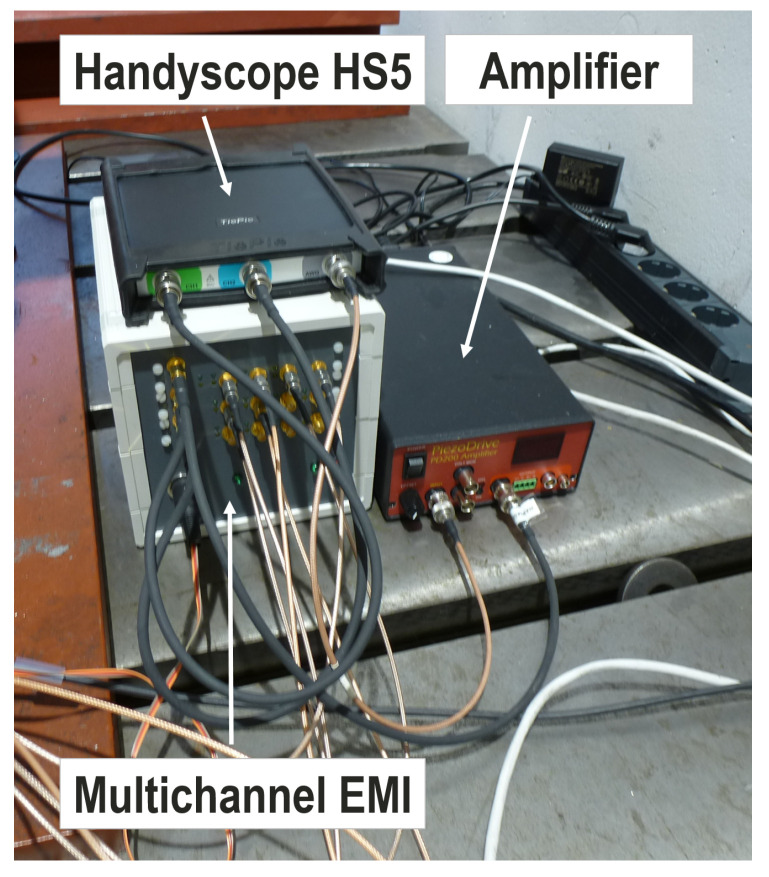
Photo of the electro-mechanical impedance (EMI) subsystem, including the multichannel EMI device, a Handscope HS5 for signal generation and A/D conversion and an ultrasound amplifier.
